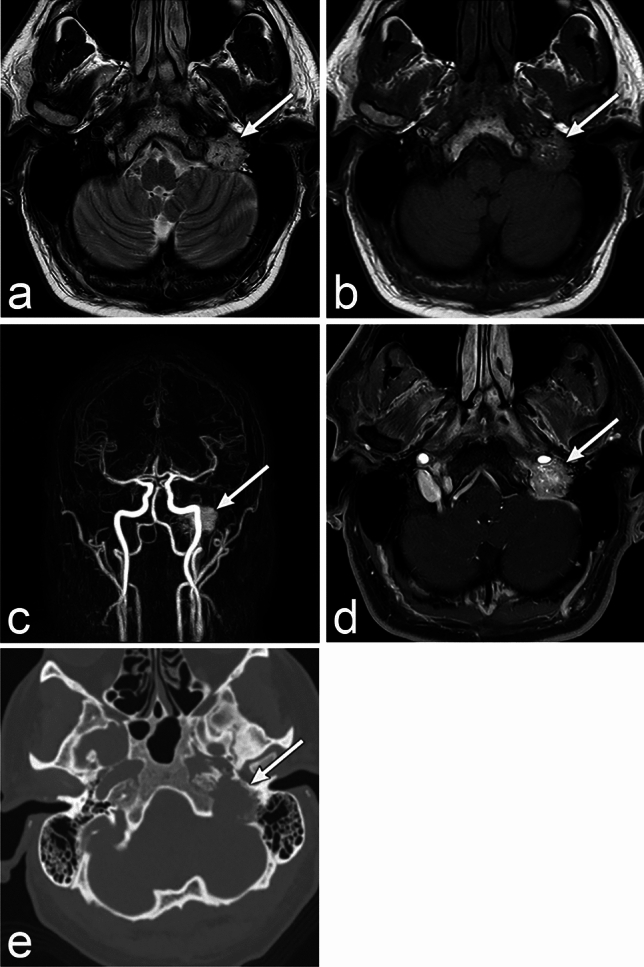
Paraganglioma of a man in his 50s with hoarseness. a–d MRI shows a well-defined mass (arrow) in the left jugular foramen. The mass shows a "salt and pepper appearance," which is a coexistence of dotted high and low signals on the T2 weighted image (WI) and T1WI. The mass shows early, marked enhancement. e CT shows enlargement of the left jugular foramen with bone erosion (arrow)
